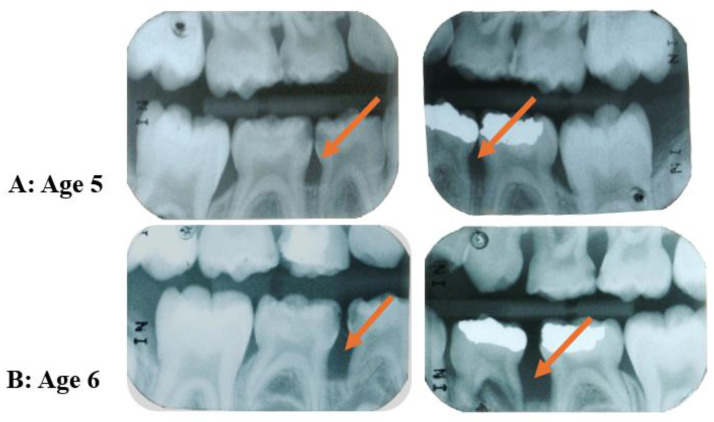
Retrospective evaluation of radiographs of a sibling of a C-MIP patient. Childhood radiographic records show initial bone loss at the first primary molars at five years old (A). The disease progressed fast and spread to the second primary molars at age 6 (orange arrows) (B). (Source of the image [16]).
